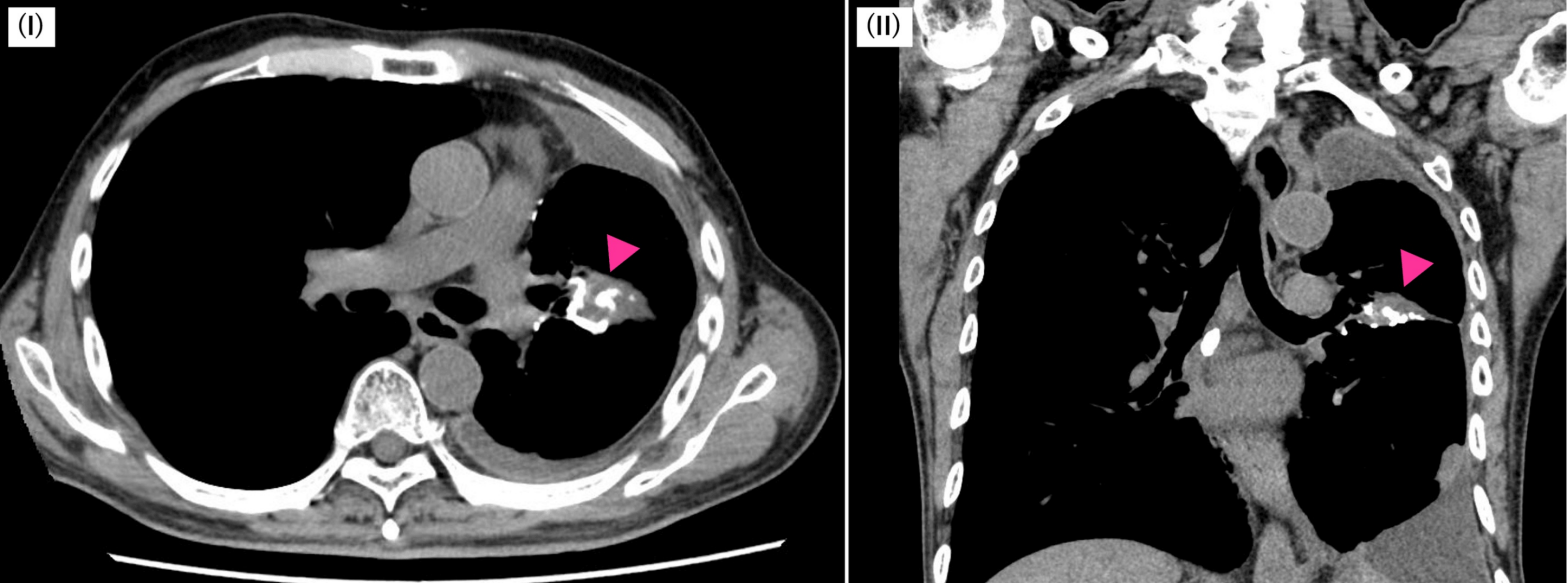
Plain chest CT. I: axial; II: coronal CT scan one month after radiotherapy shows a reduced mass of 30 x 21 x 17 mm in the left upper lobe lung around postoperative petz (arrowhead). Window center 30, window width 300.
